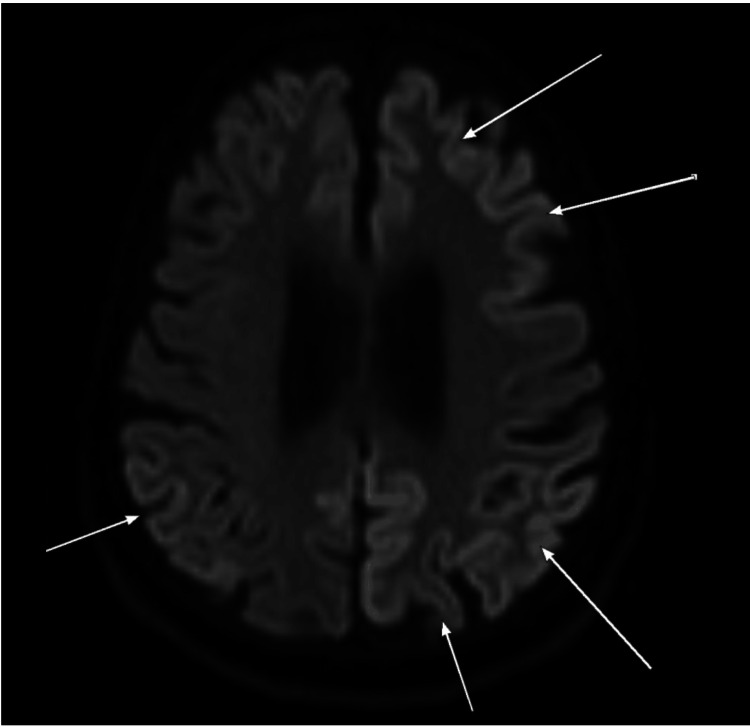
Diffusion-weighted imaging (DWI) demonstrating diffusion restriction characterized by cortical ribboning in the left frontal and bilateral parietal lobe cortices (long arrow).
